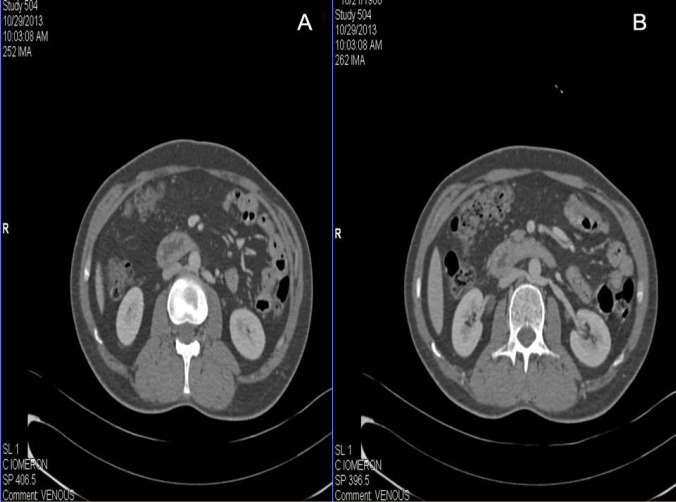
Axial images of the venous phase of CT renal angiography showing two left renal veins draining into the IVC with retro-aortic course
